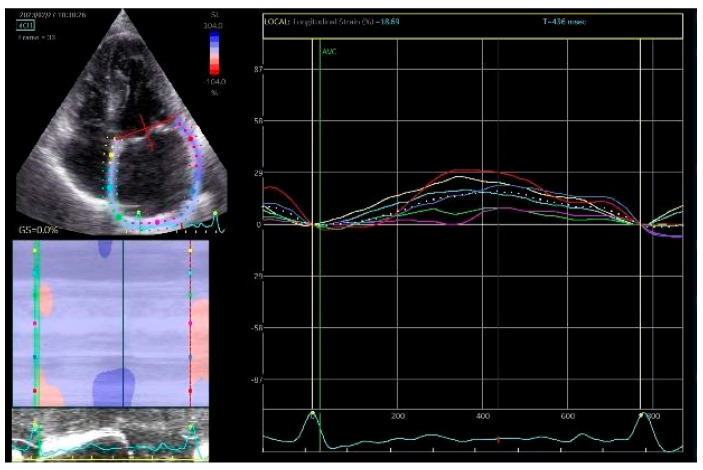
Assessment of LA strain in apical 4-chamber section.
